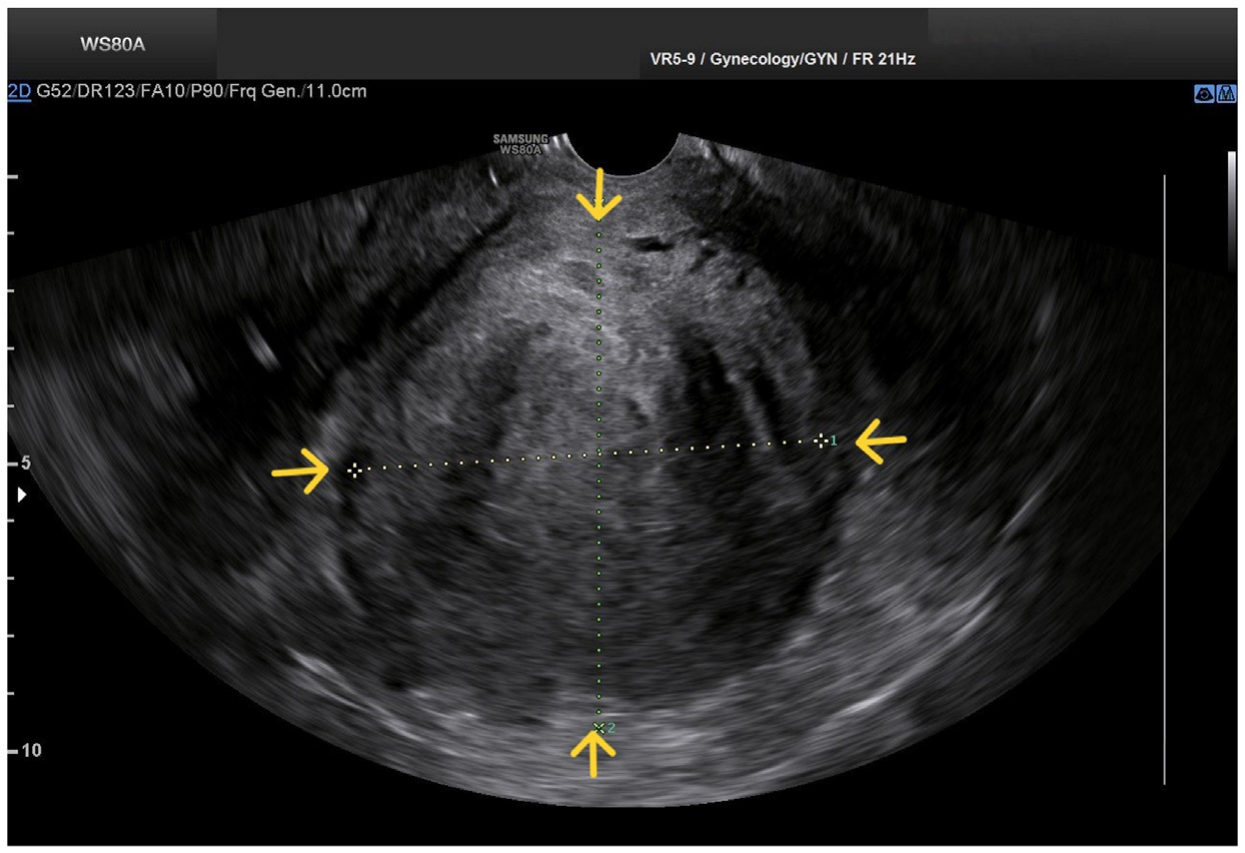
Transvaginal ultrasound image of a large prolapsed pedunculated submucosal uterine leiomyoma: The pelvic mass is clearly depicted (yellow arrows), without clear imaging of the adjacent anatomical structures.
